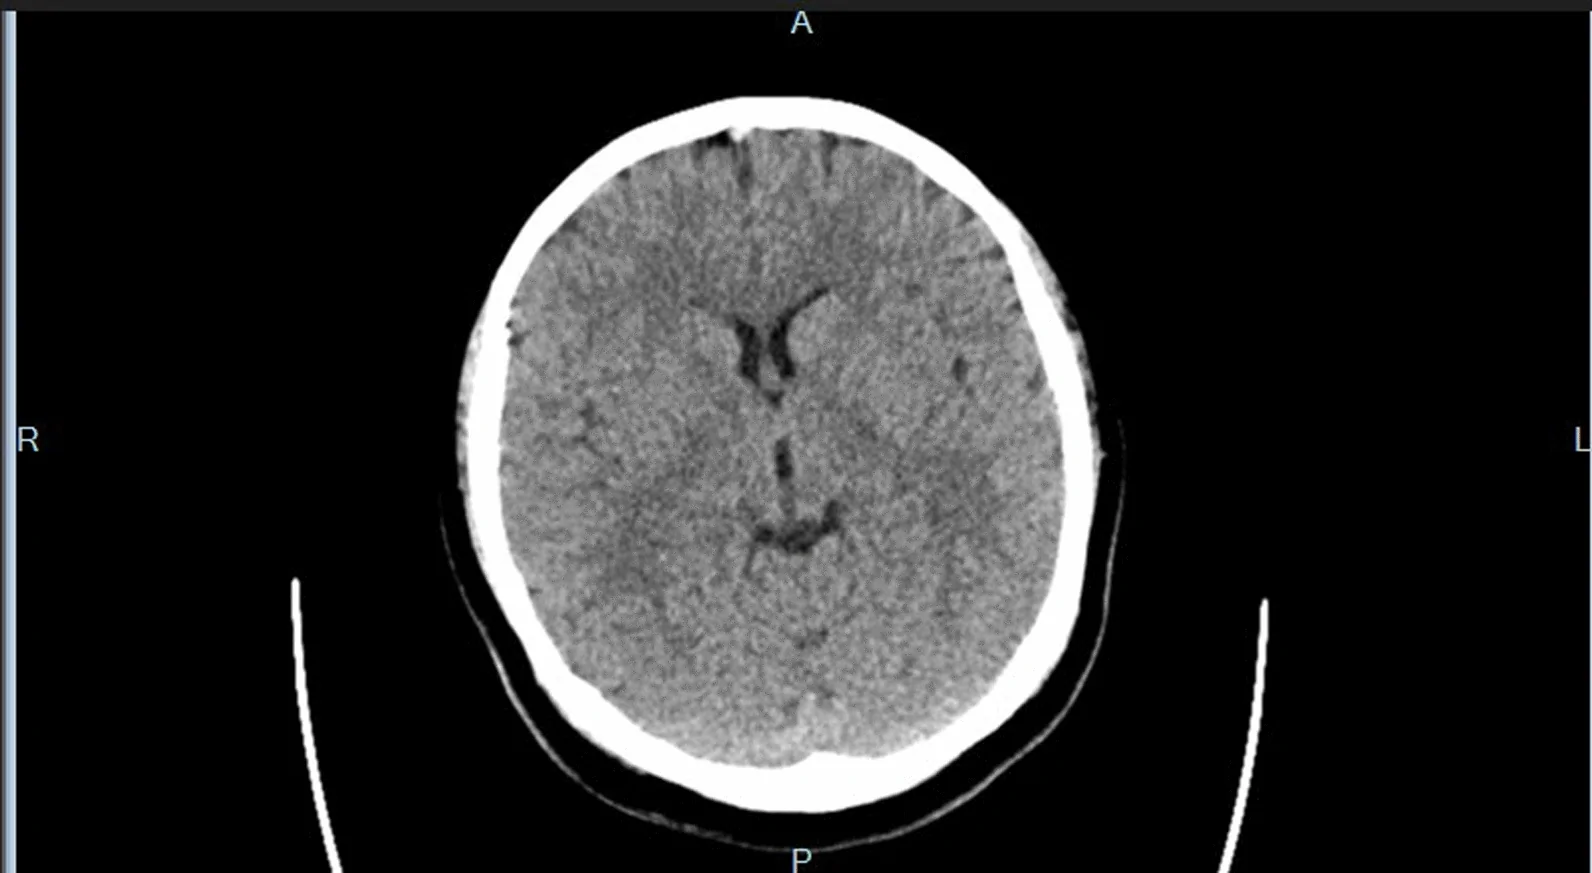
Coronal CT brain (non-contrast) showing no pituitary enlargement, mass effect, or suprasellar lesion suggestive of structural pathology
